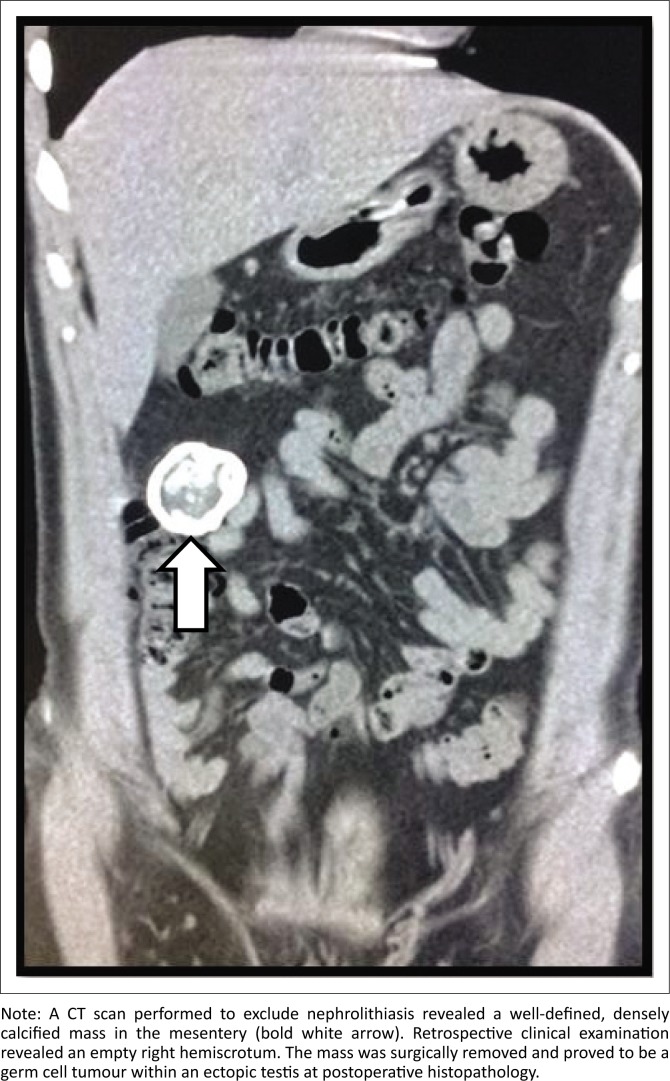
Coronal non-contrast computed tomography abdomen of a 35-year-old male who presented to the emergency department with right flank pain.
